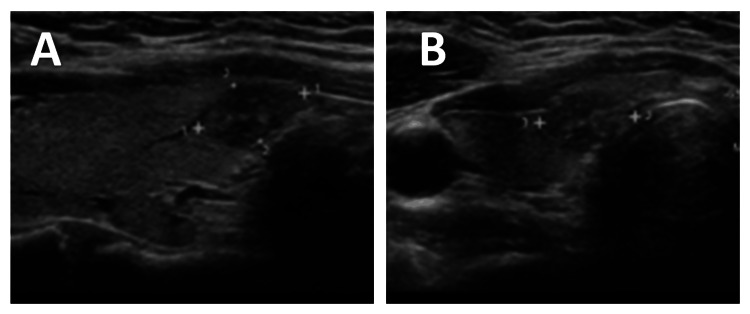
Surveillance thyroid ultrasound demonstrating interval nodule growth (A) Transverse grayscale ultrasound image of the right thyroid lower pole showing interval enlargement of the previously identified nodule with a mildly heterogeneous and predominantly hypoechoic appearance. (B) Longitudinal grayscale view of the same nodule confirming interval growth compared with prior imaging.
